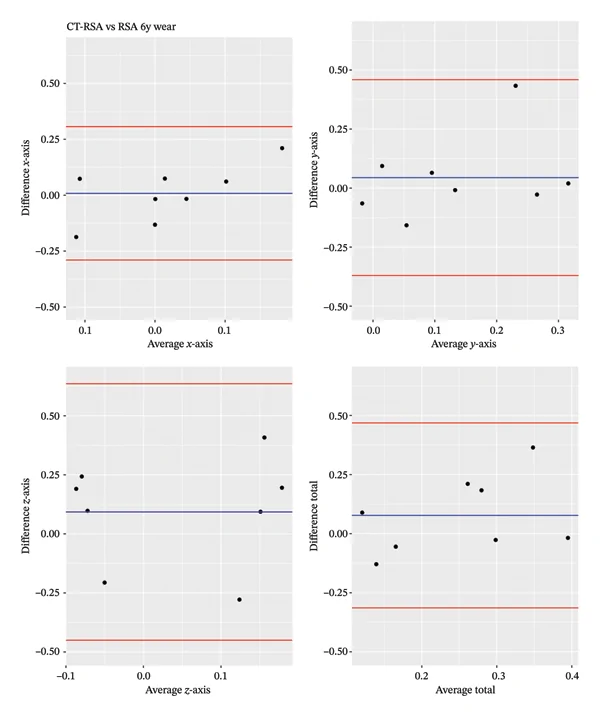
Agreement between CT‐RSA and RSA for wear measurements in *x*, *y*, and *z* translations and total translation (MTPM for RSA) at 6 years postoperatively. The blue line represents the mean difference, and the red lines represent the upper and lower limits of agreement (mean difference ± 2.365 × SD).
